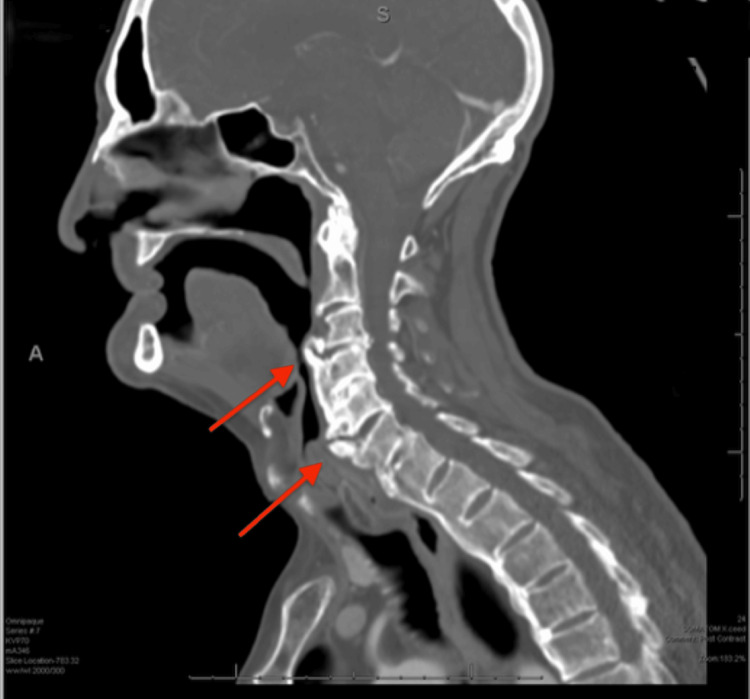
Preoperative contrast-enhanced CT of the neck demonstrating a markedly prominent C5/C6 osteophyte compressing the left pyriform fossa. No abnormal lymph nodes were identified. Original CT image from Royal Hobart Hospital, Australia, 2025, reproduced with permission from Royal Hobart Hospital Radiology Department
